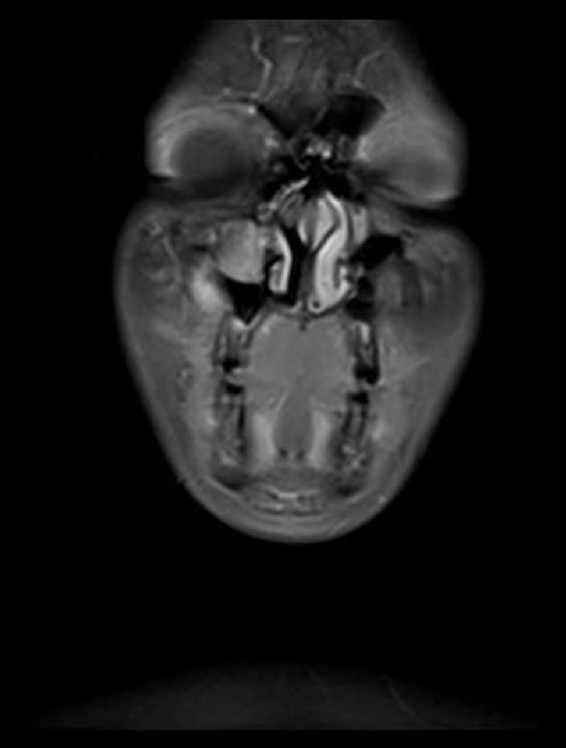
A coronal MRI of the sinuses revealed a mass in the right maxillary sinus.
